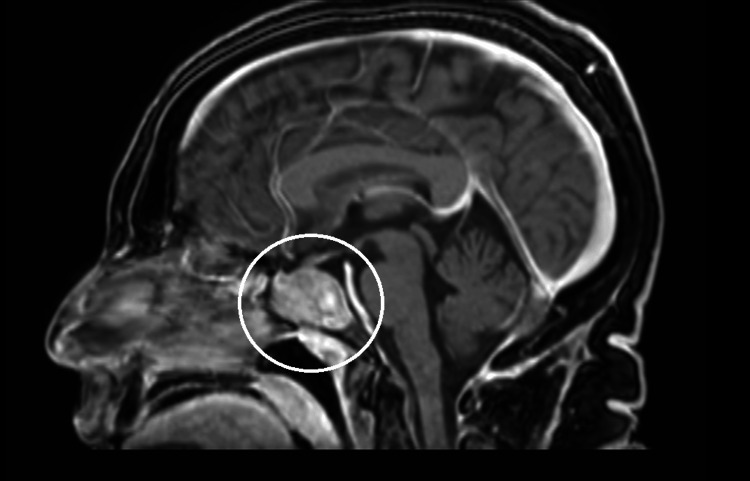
MRI of the pituitary gland, with the circle indicating the macroadenoma of the pituitary gland (sagittal view)
